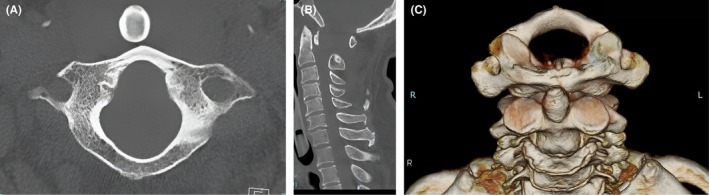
Upper cervical spine CT findings. (A) Axial image showing complete posterior dislocation of the anterior arch of the atlas behind the odontoid process, without definitive fracture. (B) Sagittal image clearly confirming the positional relationship: The anterior arch of the atlas lies entirely posterior to the odontoid process. The red arrow on the 3D volume‐rendered CT image marks dislocation at the same location. (C) Three‐dimensional reconstructed image providing a comprehensive spatial visualization of the posterior dislocation of the atlas relative to the axis.
